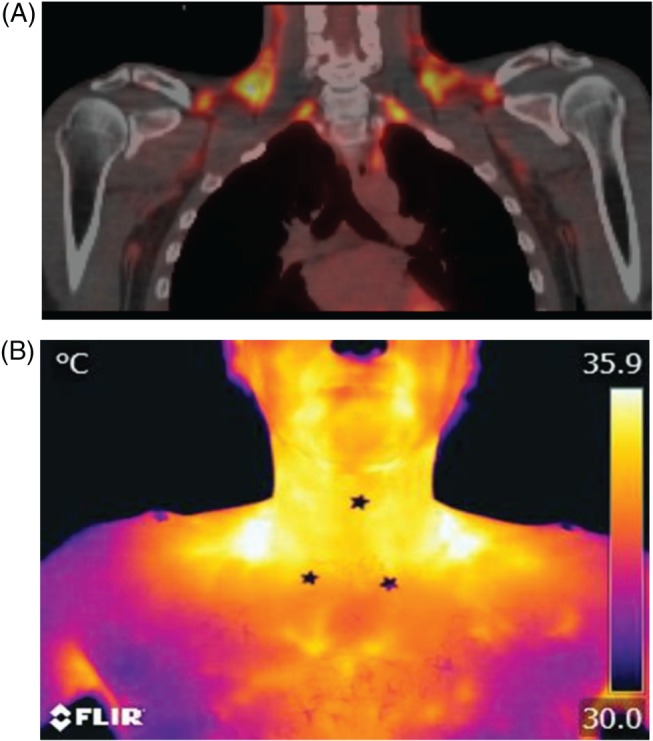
Examples of positron emission tomography (PET)/CT and thermal images from the same subject at the end of a cooling vest visit. (A) Fused ^1^
